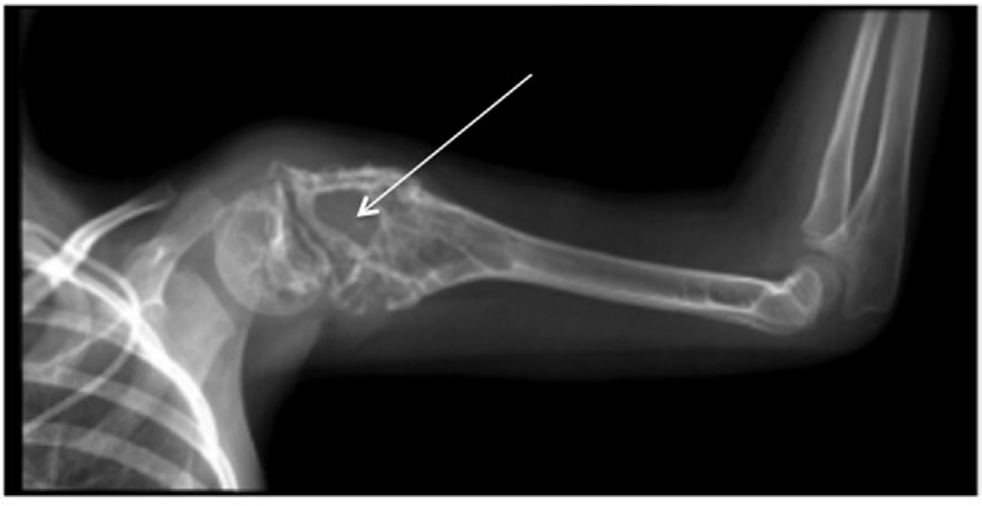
X-ray of typical severe osteolytic lesions in a Ewing sarcoma patient (arrows: severe osteolytic lesions).
